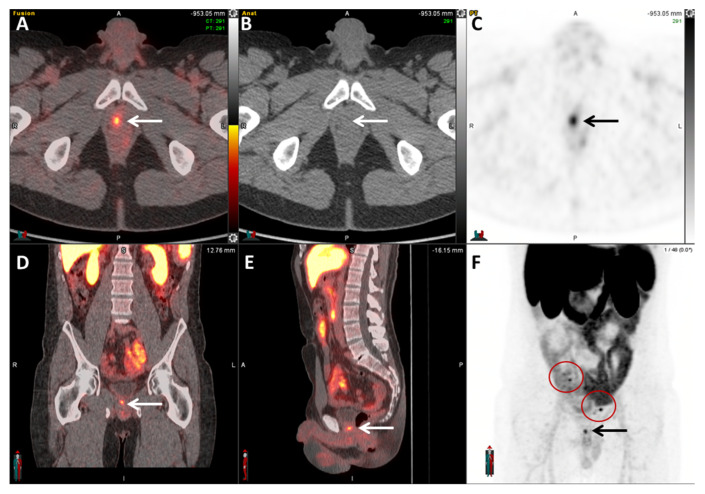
A 53-year-old man with biochemically recurrent prostate cancer with PSA of 0.4 ng/mL. Axial fused PET/CT (A), axial CT (B), axial PET (C), coronal PET (D), sagittal PET (E), and MIP images (F) demonstrate a focal area of increased ^18^F-PSMA-1007 uptake at the right aspect of the bladder neck (arrows, SUVmax = 7.3). The low urinary excretion of ^18^F-PSMA-1007 facilitates the evaluation of the prostatectomy bed. MIP images demonstrate additional foci of increased uptake in the pelvis bilaterally (circles), compatible with nodal metastases.
